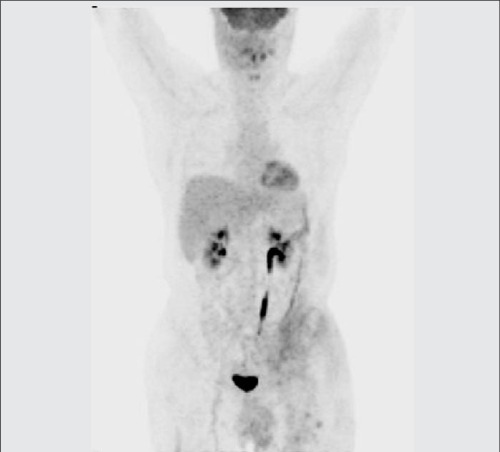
MIP whole body image showed non-homogeneous FDGuptake (SUV_max_:3.0) on the left lower side of anterior abdominalwall which was considered as secondary to the treatment
